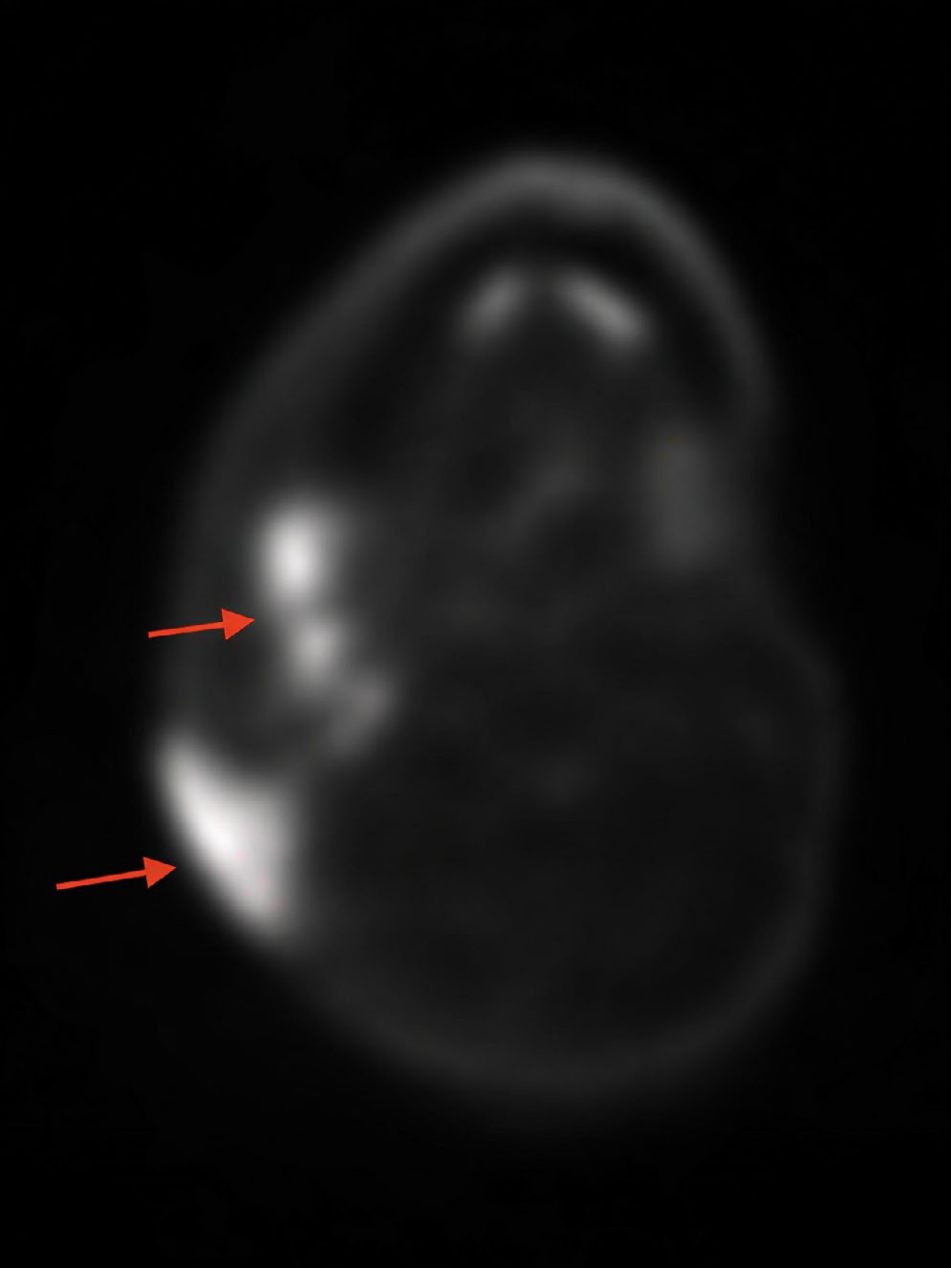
Neck PET/CT showing local soft tissue invasion and prominent right cervical lymph nodes with increased uptake concerning for metastasis (red arrows).
